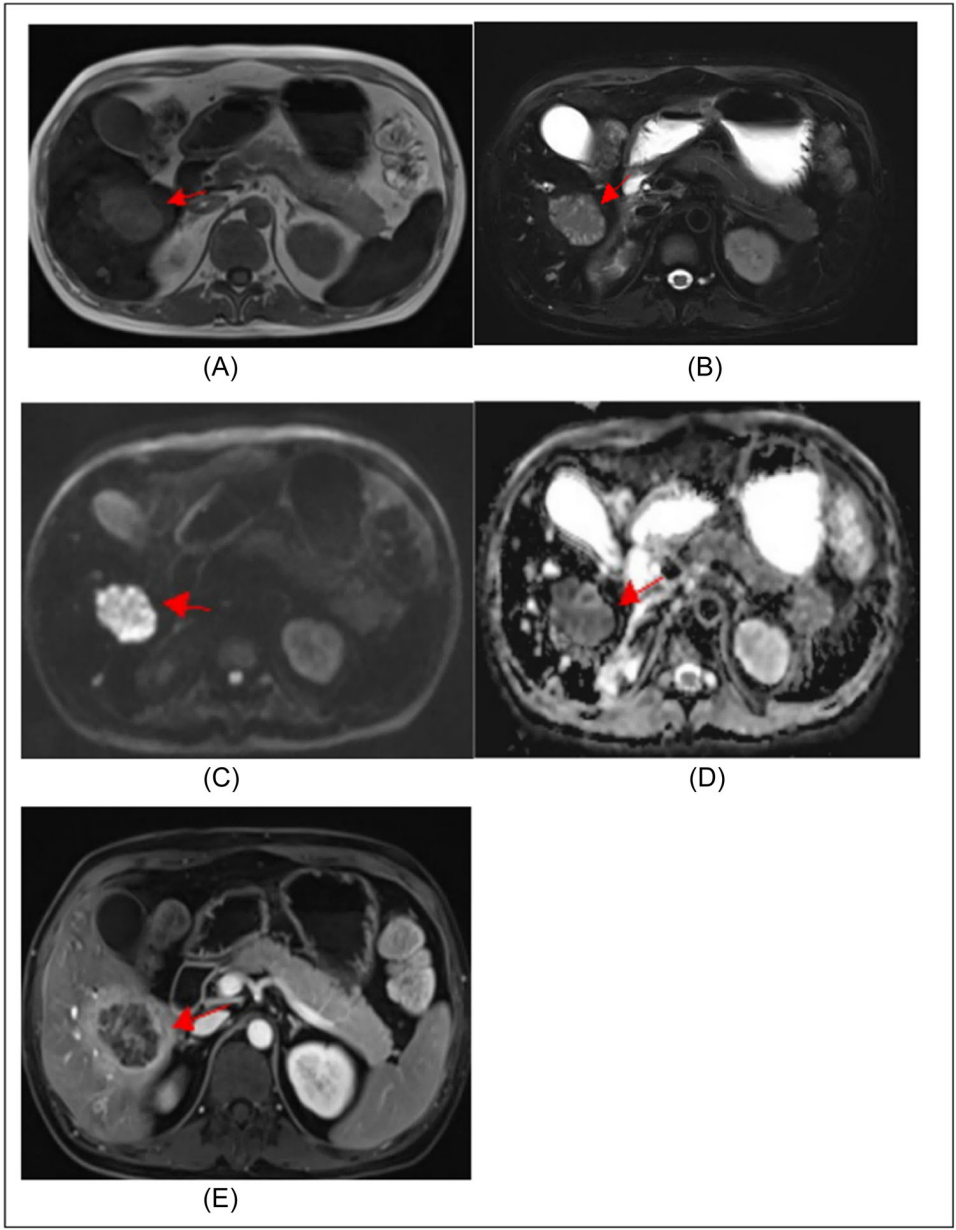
Representative MR images in a 30‐year‐old man patient with hepatic fungal infection. The images of the lesion on T1WI (A), T2WI (B), DWI (C), ADC map (D), and contrast enhancement (E).
